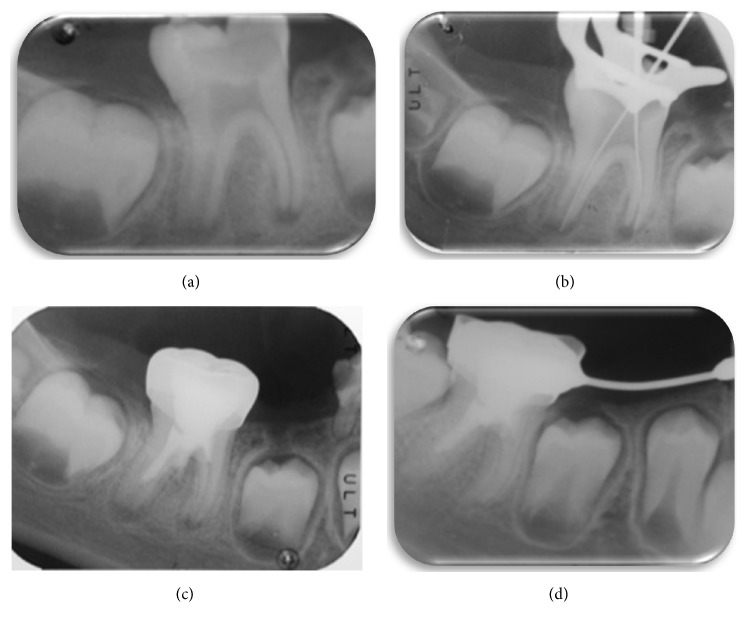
(a) First visit showing occlusal caries on tooth 46 extending to the pulp chamber, with open apices. (b) Determination of working length. (c) End of revascularization therapy, having placed MTA and metal crown. (d) Follow-up radiograph. Apical closure of both roots is shown and radiotranslucencies have disappeared. A complication associated with revascularization is obliteration of the root canals that is shown here.
